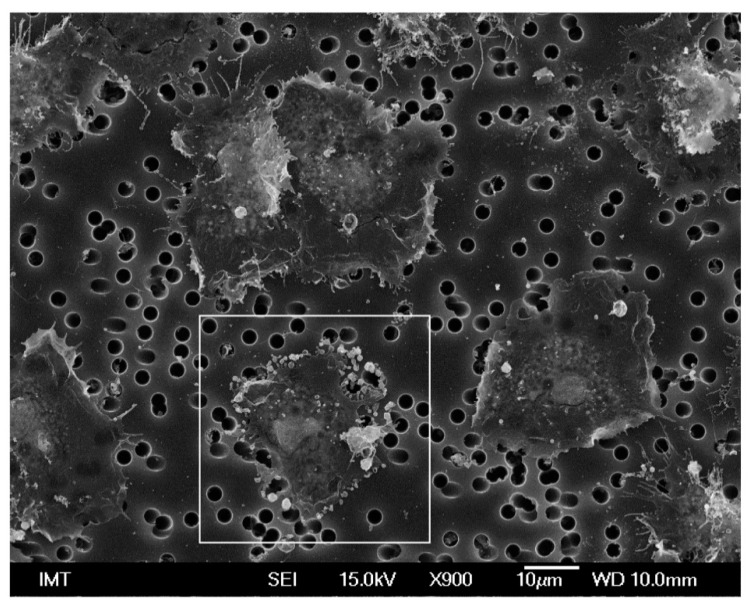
SEM images of hemocytes of *M. galloprovincialis* incubated for 30 min with PVP-AuNPs (10 µg/mL) in ASW suspension. In the framed cell, the presence of PVP-AuNPs was investigated using Energy-dispersive X-ray (EDX) analysis, and the details are presented in Figure S2.
